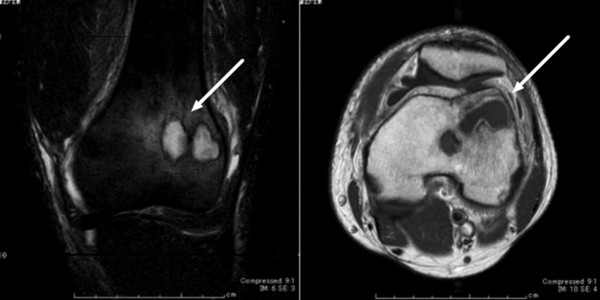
MRI of the left knee; longitudinal scan on T2-weighted image (left panel), horizontal scan on enhanced T1-weighted image (right panel). Polycystic bone abscess lesions were detected in the end of the left femur.
